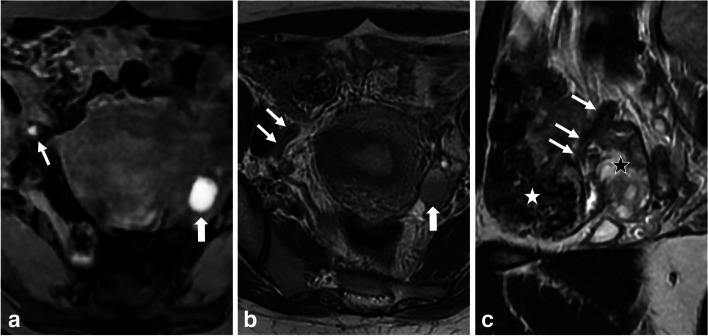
Normal appendix filled with feces in a 34-year-old woman with chronic pelvic pain and medical history of endometriosis referred for infertility issues. a Axial T1-W fat-suppressed MR image shows a T1-hyperintense “spot” in the right lateral pelvic side (thick arrow). b Axial and (c) sagittal T2-W MR images show that the T1-hyperintense “spot” is located in a tubular structure (thin arrows) referring to the appendix running over the right ovary (black star), consistent with endoluminal feces concretion (white star). Note the presence of a left endometrioma with T2 shading (thick arrows in a and b)
